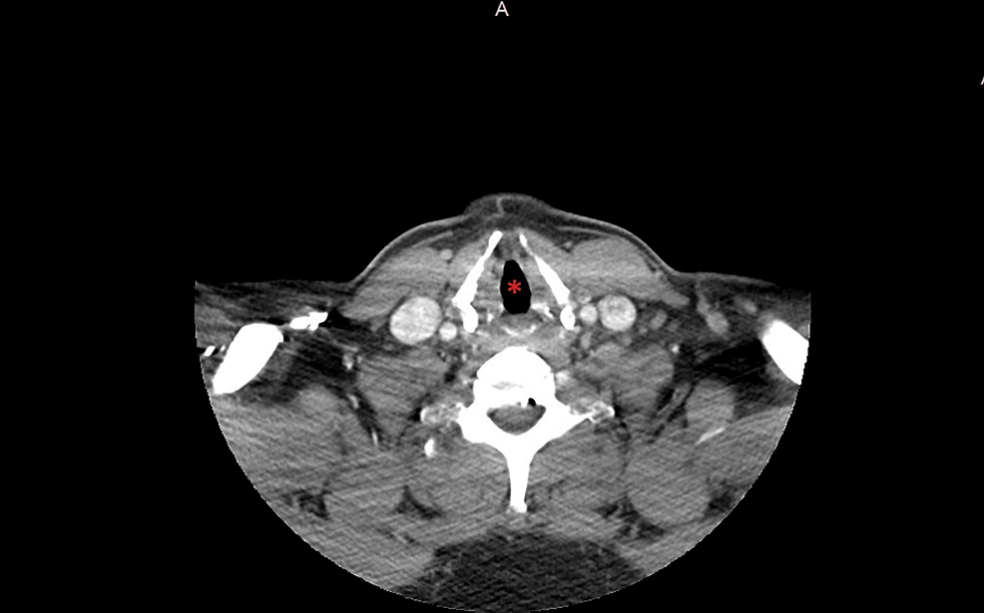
CT neck showing the patient's airway. The red asterisk highlights a patent airway, with no edema or airway compromise.
